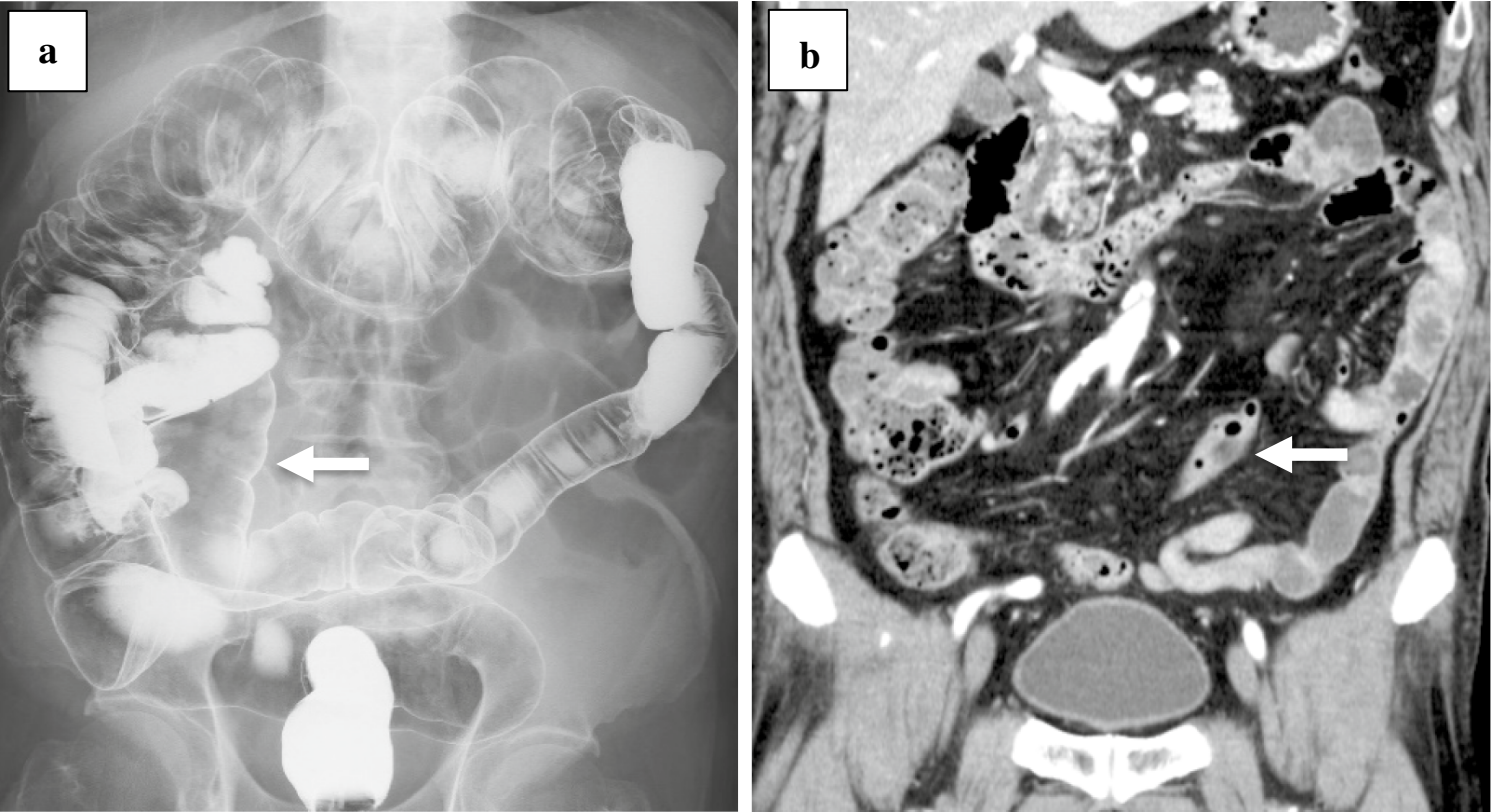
Case 1. a BE revealed the marked displacement of the descending colon with paracecal fixation (white arrow) and the sigmoid colon was on the right side of the abdomen. b An enhanced CT showed the descending colon (white arrow)
